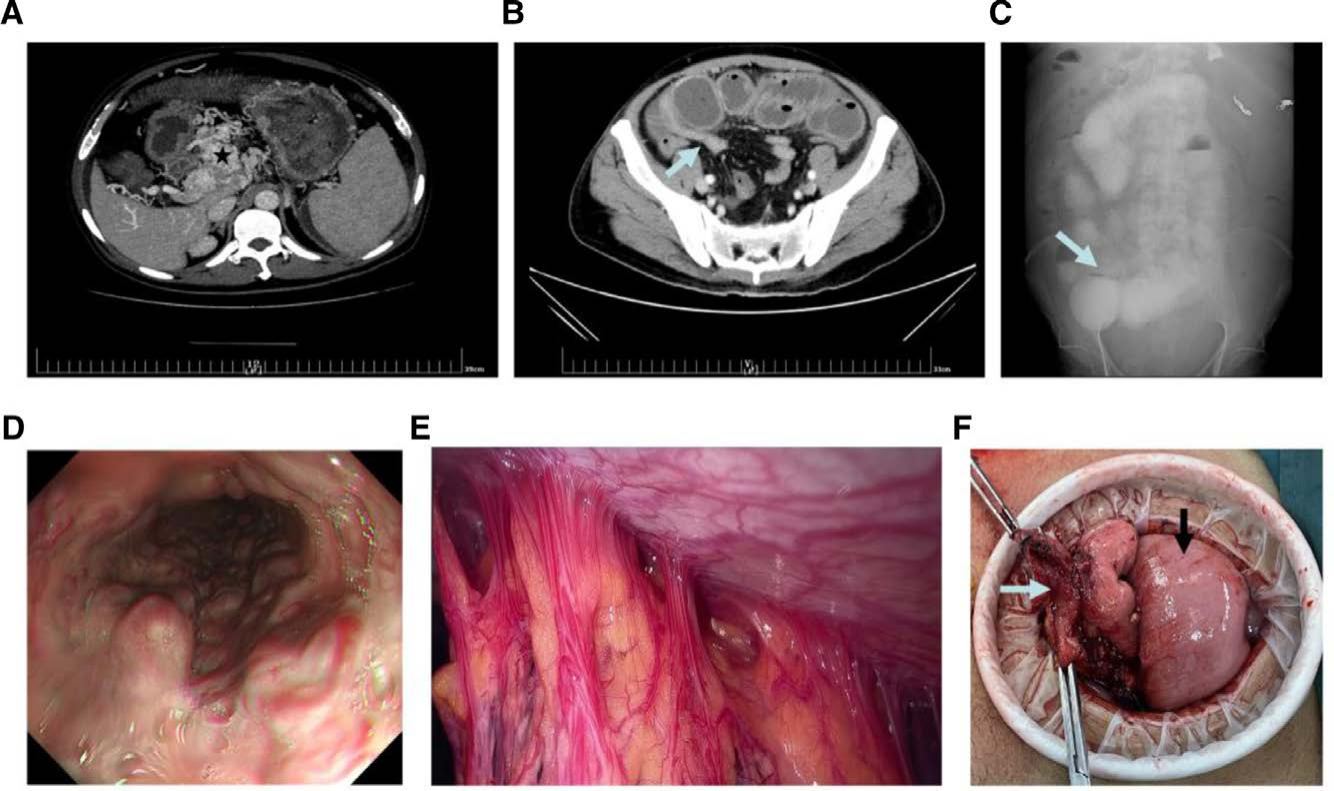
(A) Cavernous transformation of the portal vein, multiple tortuous vascular shadows around the portal vein, around the stomach and the splenic hilum (black pentagram). (B) Local intestinal wall thickened, intestinal lumen narrowed (blue arrow), and proximal small intestine dilated. (C) Gastrointestinal radiography revealed local stenosis of the ileum with a beak sign (blue arrow). (D) Gastroscopy revealed severe esophagogastric varices. (E) Extensive adhesion of bowel, mesentery, and abdominal wall was found during the operation. (F) Narrow section of the ileum (blue arrow), and proximal dilated small intestine (black arrow).
